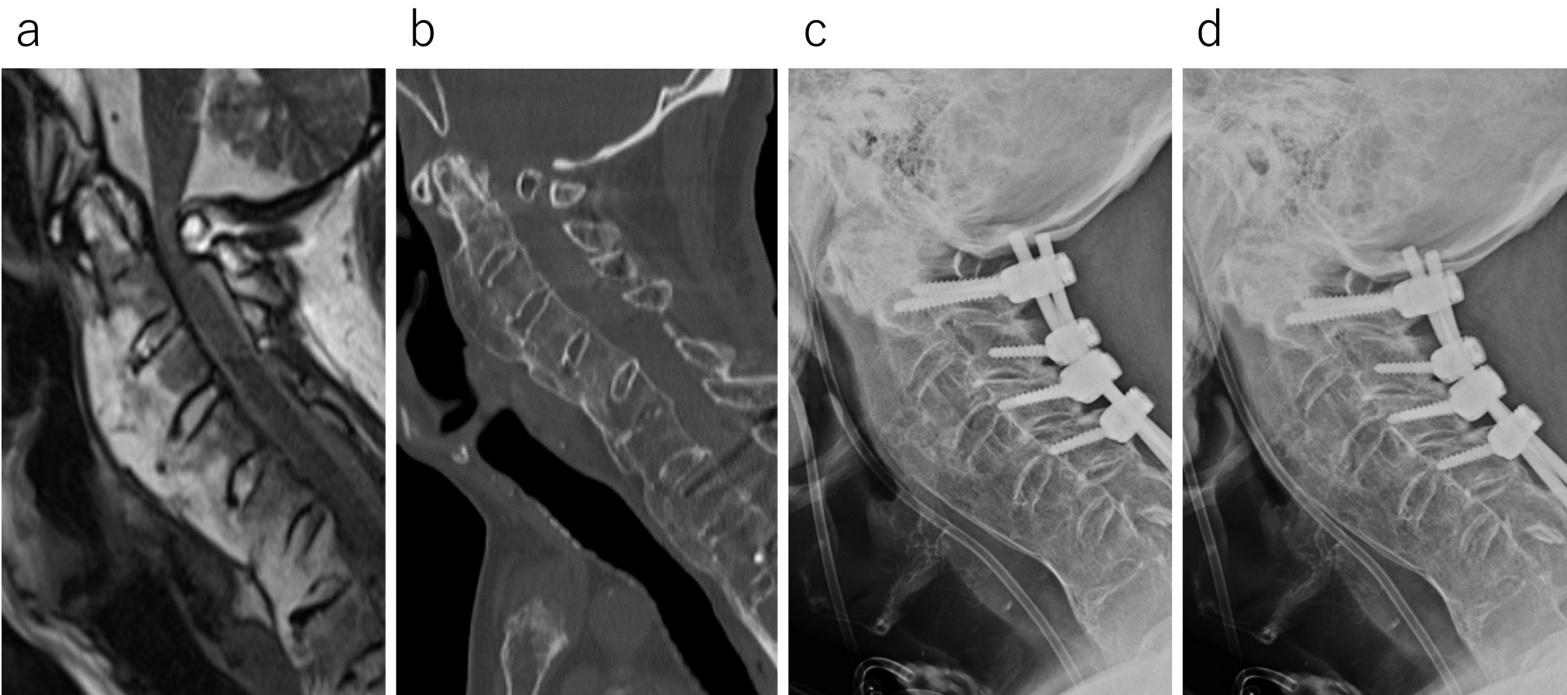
Imaging tests are recommended for the initial consultation. Sagittal T2-weighted MRI demonstrates substantial spinal cord compression at C1/2 (a). The atlantodental interval measures approximately 1 mm on the sagittal CT scan (b). X-ray images of anteflexion (c) and retroflexion (d) similarly reveal minimal instability at C1/2.
